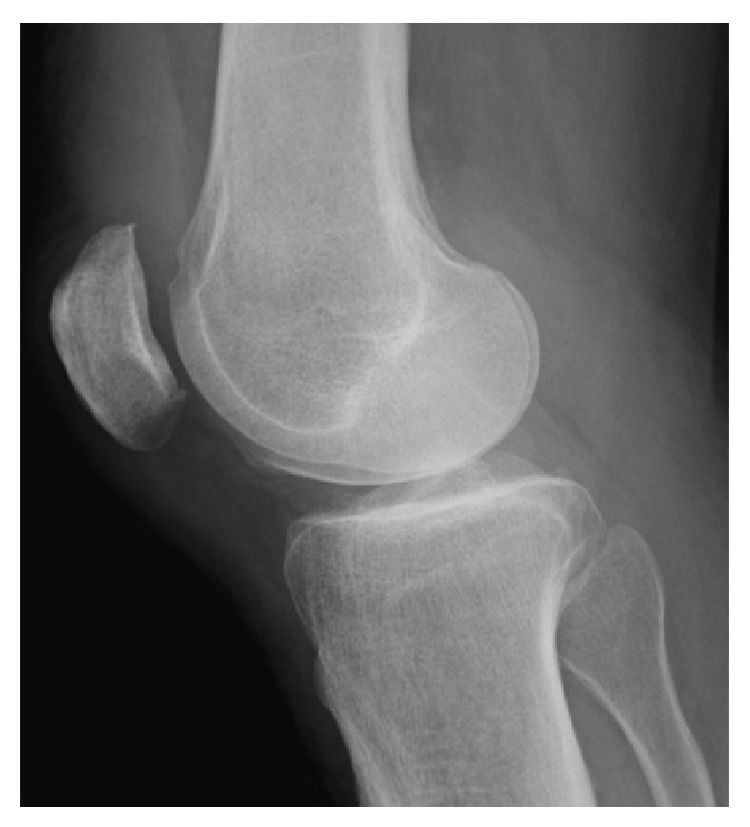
Lateral plain radiograph. Osteophyte formation was observed in the upper pole of the patella.
